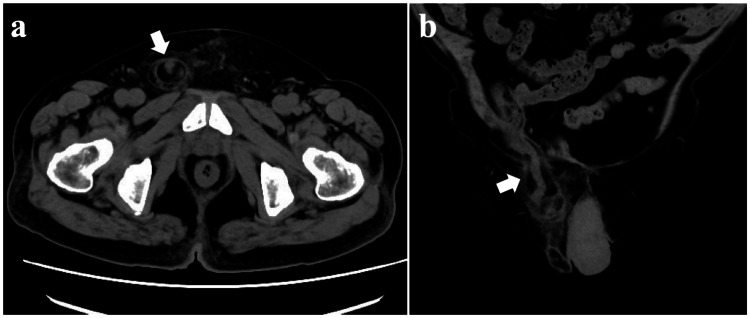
Abdominal CT scan one month ago: (a) axial view and (b) coronal view. Abdominal CT reveals the right inguinal hernia containing the appendix (arrows), without any sign of inflammation. CT: computed tomography.
